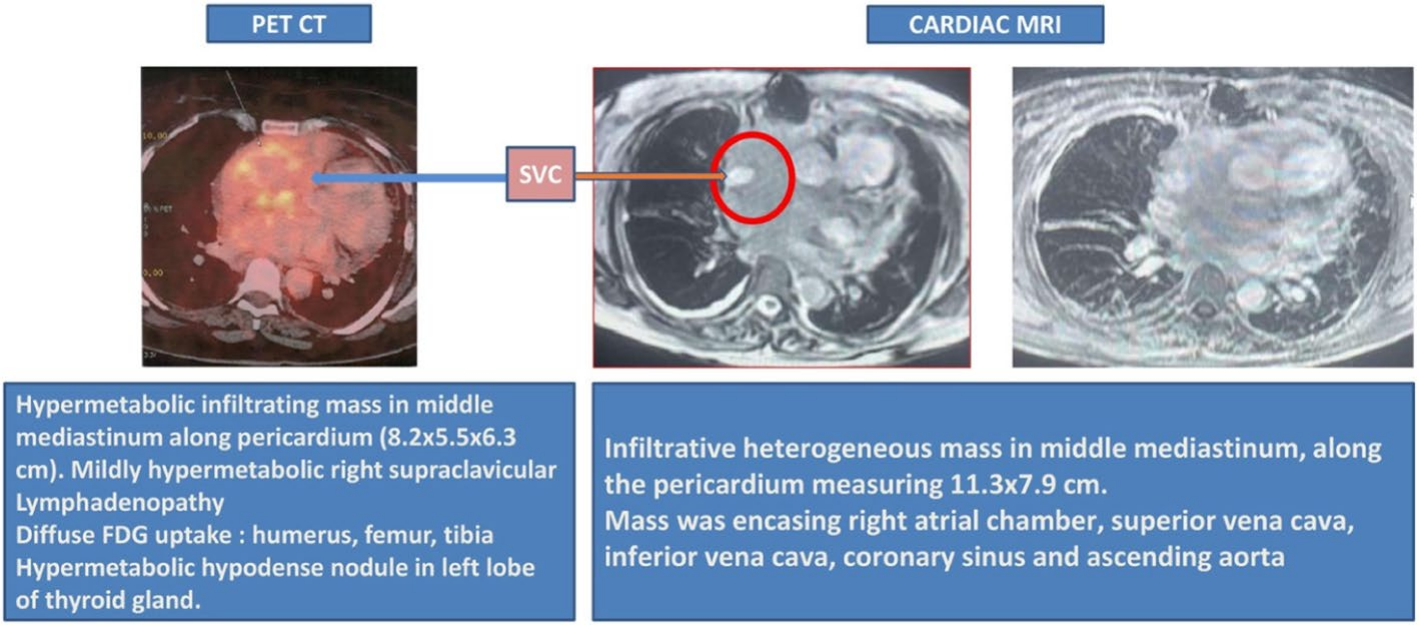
PET-CT and cardiac MRI showing a huge mass in the middle mediastinum along the pericardium at diagnosis. FDG, fluorodeoxyglucose; MRI, magnetic resonance imaging; PET/CT, positron emission tomography/computed tomography
